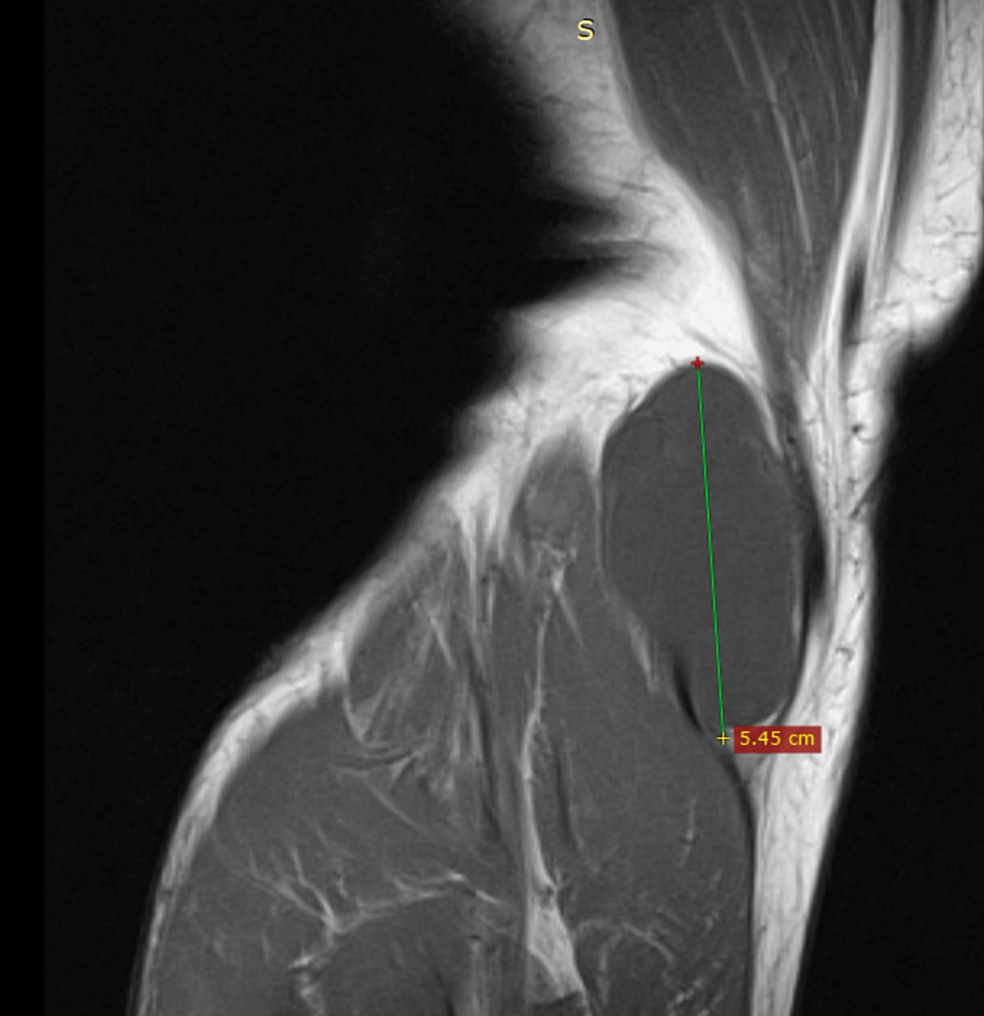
Coronal T2-weighted image that shows a low-intensity signal cyst located near the gastrocnemius medial head. Selected sample MRI studies demonstrate the length of the cyst in relation to adjacent anatomical structures.
